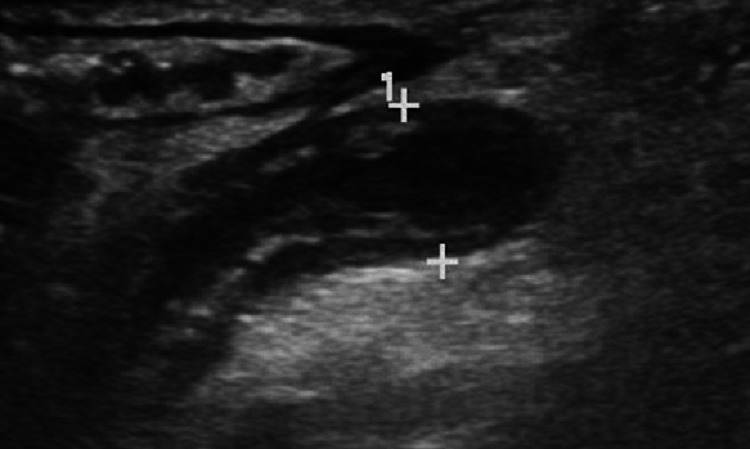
Ultrasonography image of the appendix and surrounding tissues.
